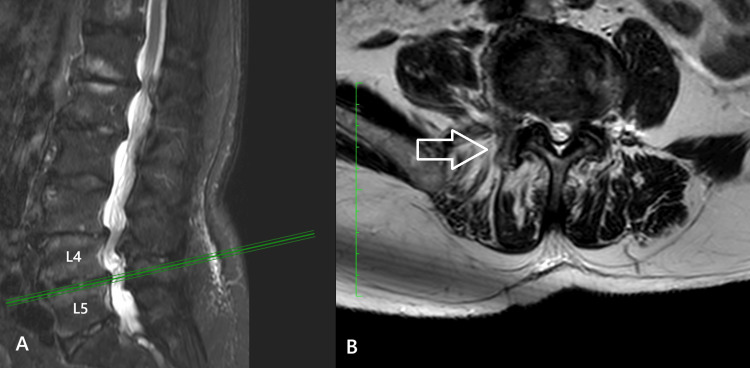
(A) T2-sagittal view and (B) T2-axial MRI views of the lumbar spine in fourth case. At the L4-L5 level, the arrows with the white outline indicate the right facet subluxation.
